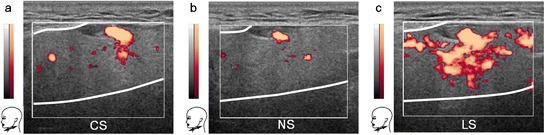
Duplex sonograms of the salivary glands under different stimulation types. The sonograms of a healthy person (male, 47 years) show relative changes in blood perfusion of the submandibular gland induced by chewing-gum stimulation (a), non-stimulation (b), and lemon-juice stimulation (c). Blood flow information is superimposed in colour onto the conventional anatomical gray-scale image. The thick white lines within the thin white rectangles indicate the approximate glandular boundaries. *The chewing-gum stimulation, non-stimulation, and lemon-juice stimulation are designated by CS, NS, and LS, respectively*.
